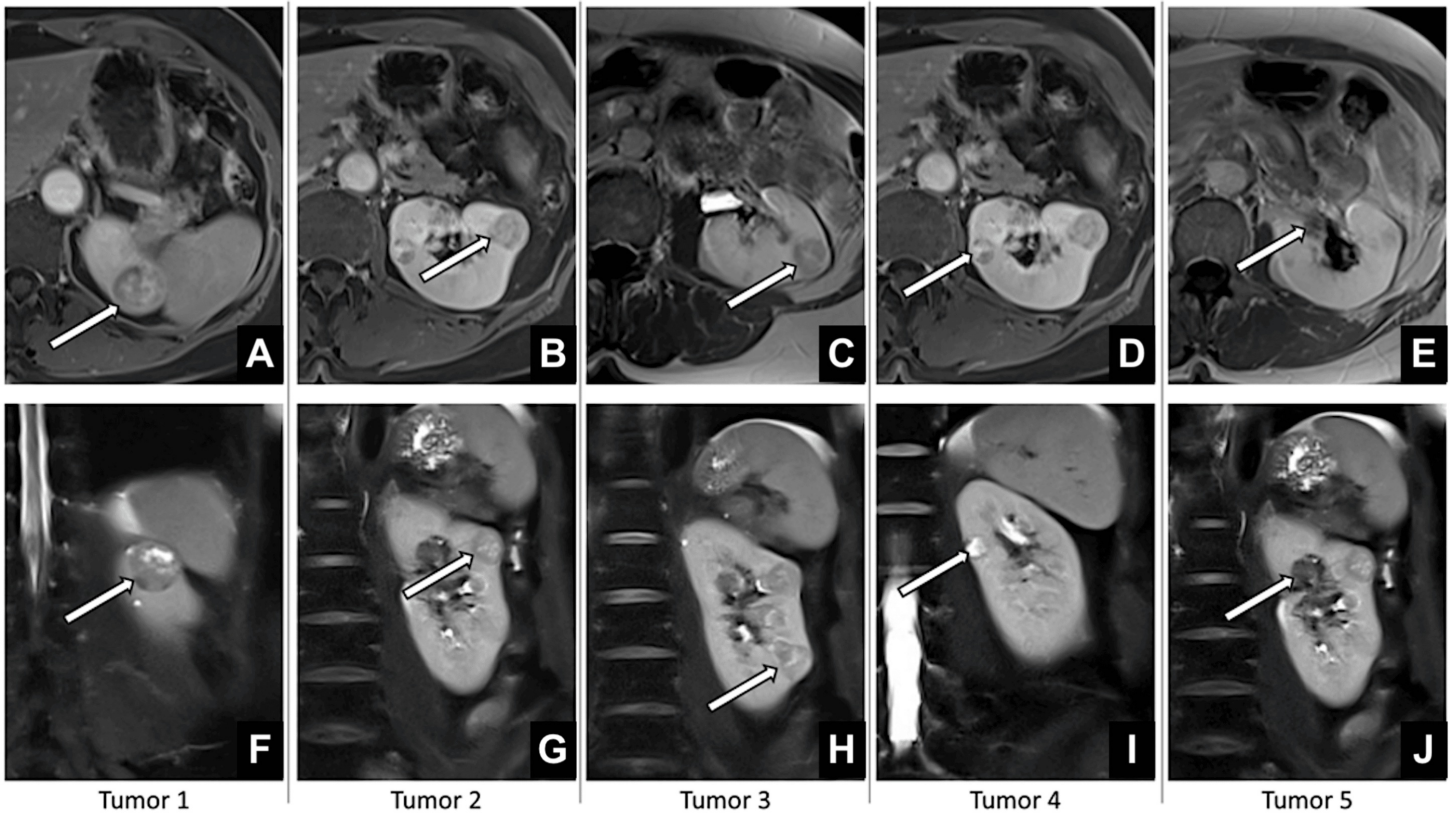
MRI of the abdomen demonstrating five renal tumors: (A-E) transverse view; (F-J) coronal view, each tumor indicated by a white arrow Image Credit: Toni Franz
